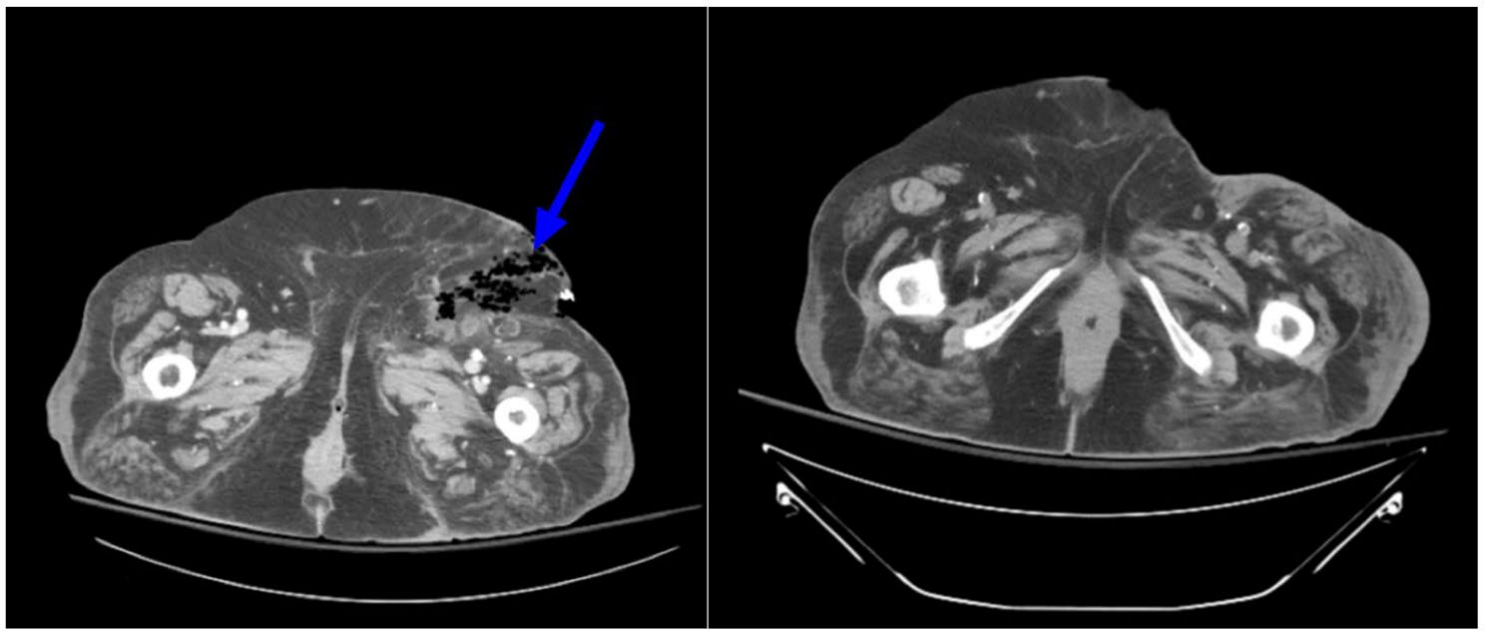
69 year old female presenting with infected groin wound. Axial CT images of the pelvis in soft tissue window demonstrate (A) fat stranding and foci of air in the left lower abdominal wall, demonstrating necrotizing fasciitis. (B) Post treatment CT pelvis in soft tissue window resolution of necrotizing fasciitis status-post debridement and repair of the left inguinal area
